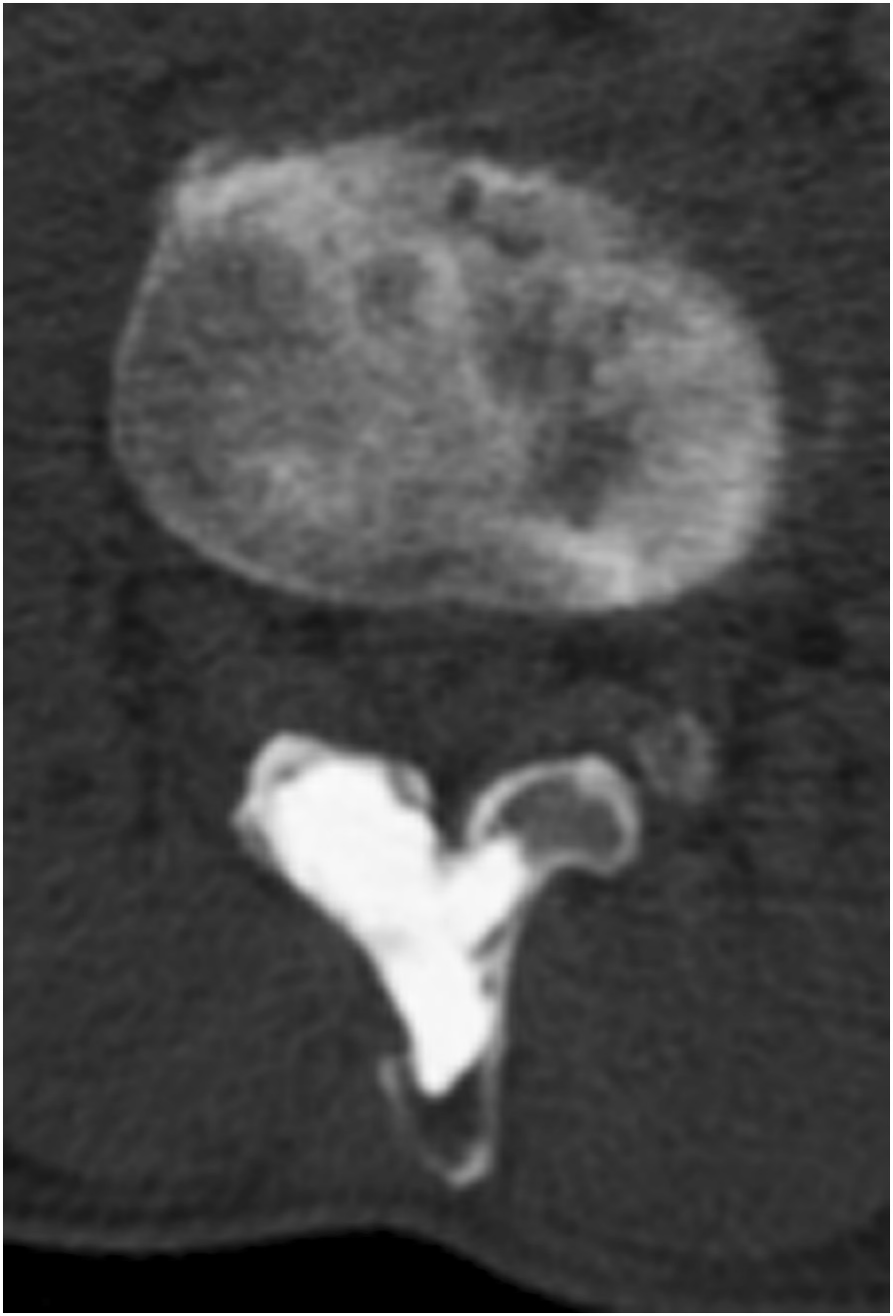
Computed tomography image three years after surgery.
